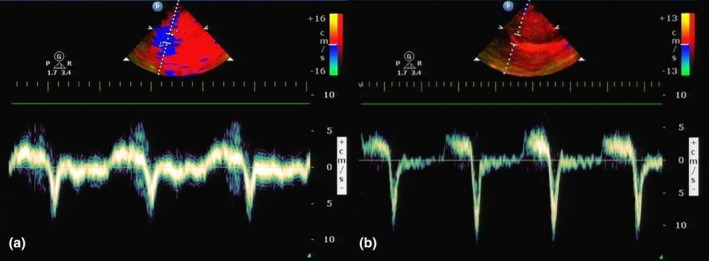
d‐TDI patterns in the two groups. (a) Patients in the successful weaning group; (b) Patients in the failure weaning group. d‐TDI, tissue Doppler imaging variables of the diaphragm.
